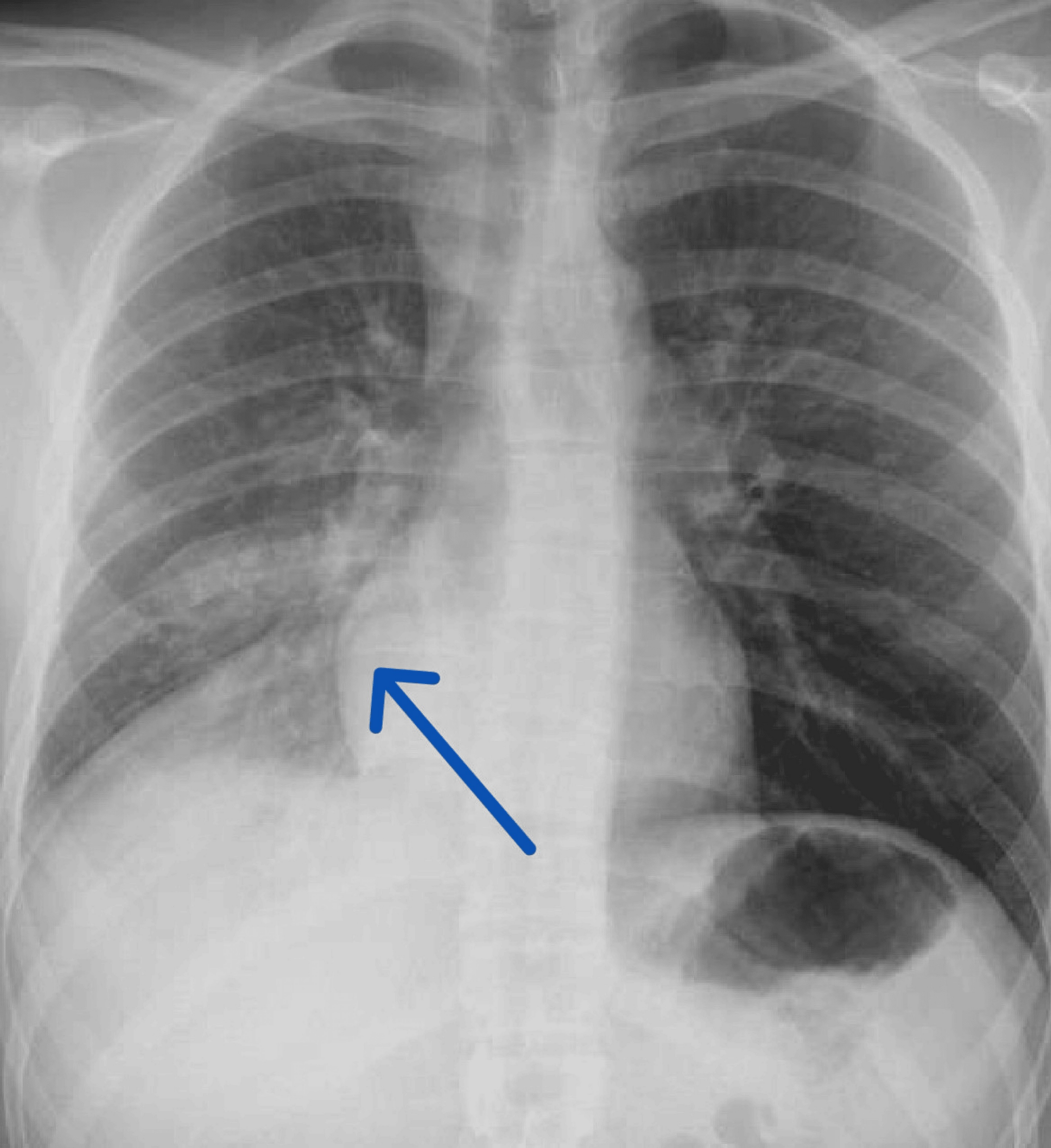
Chest X-ray (anteroposterior view) revealed right lower lobe opacity.
